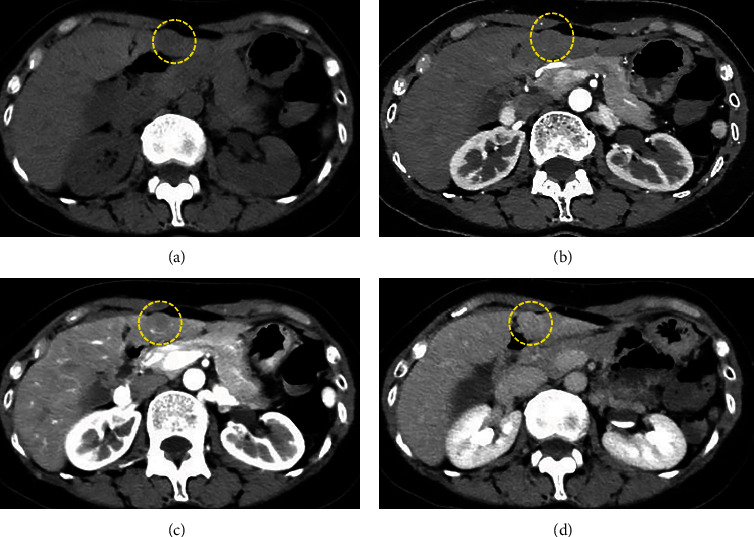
CT findings. (a) On plain CT, the mass was detected as low-density lesion. (b) The mass was not enhanced on contrast-enhanced CT in arterial phase. (c) The mass was slightly enhanced in portal phase. (d) The mass was detected as low-density lesion in delayed phase (yellow-dotted area).
